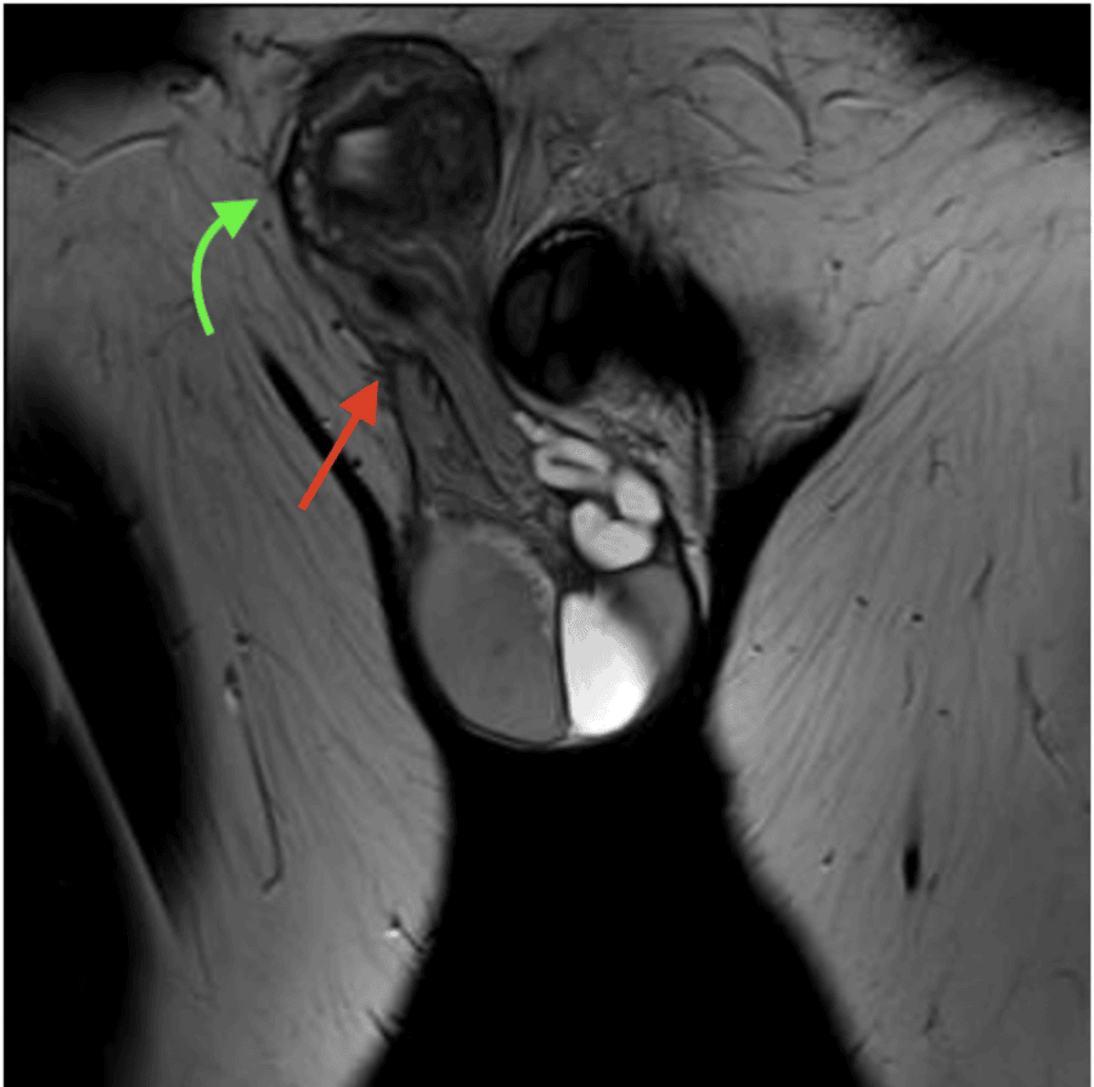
Coronal MRI image demonstrating right and left spermatic cord fusion. The red arrow indicates the fusion of the right and left spermatic cords, while the green arrow highlights a soft tissue structure at the site of the right spermatic cord near the inguinal region.
